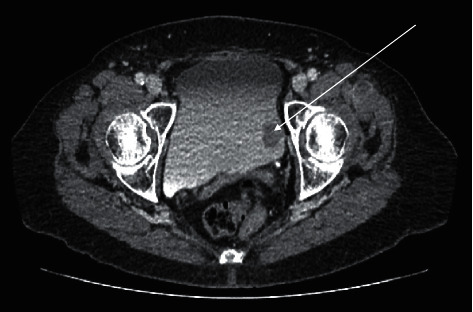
Axial section of CT urogram showing a filling defect on the left bladder wall (white arrow).
